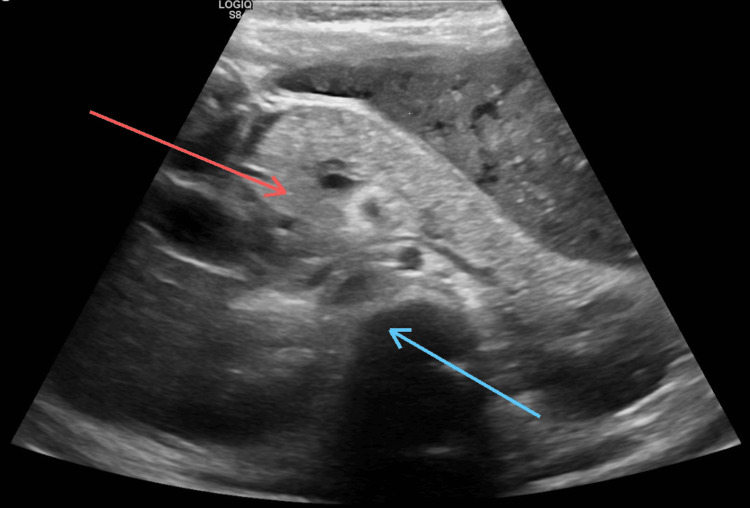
Ultrasound of the abdomen The red arrow indicates a bulky pancreas. The blue arrow indicates peripancreatic fluid.
